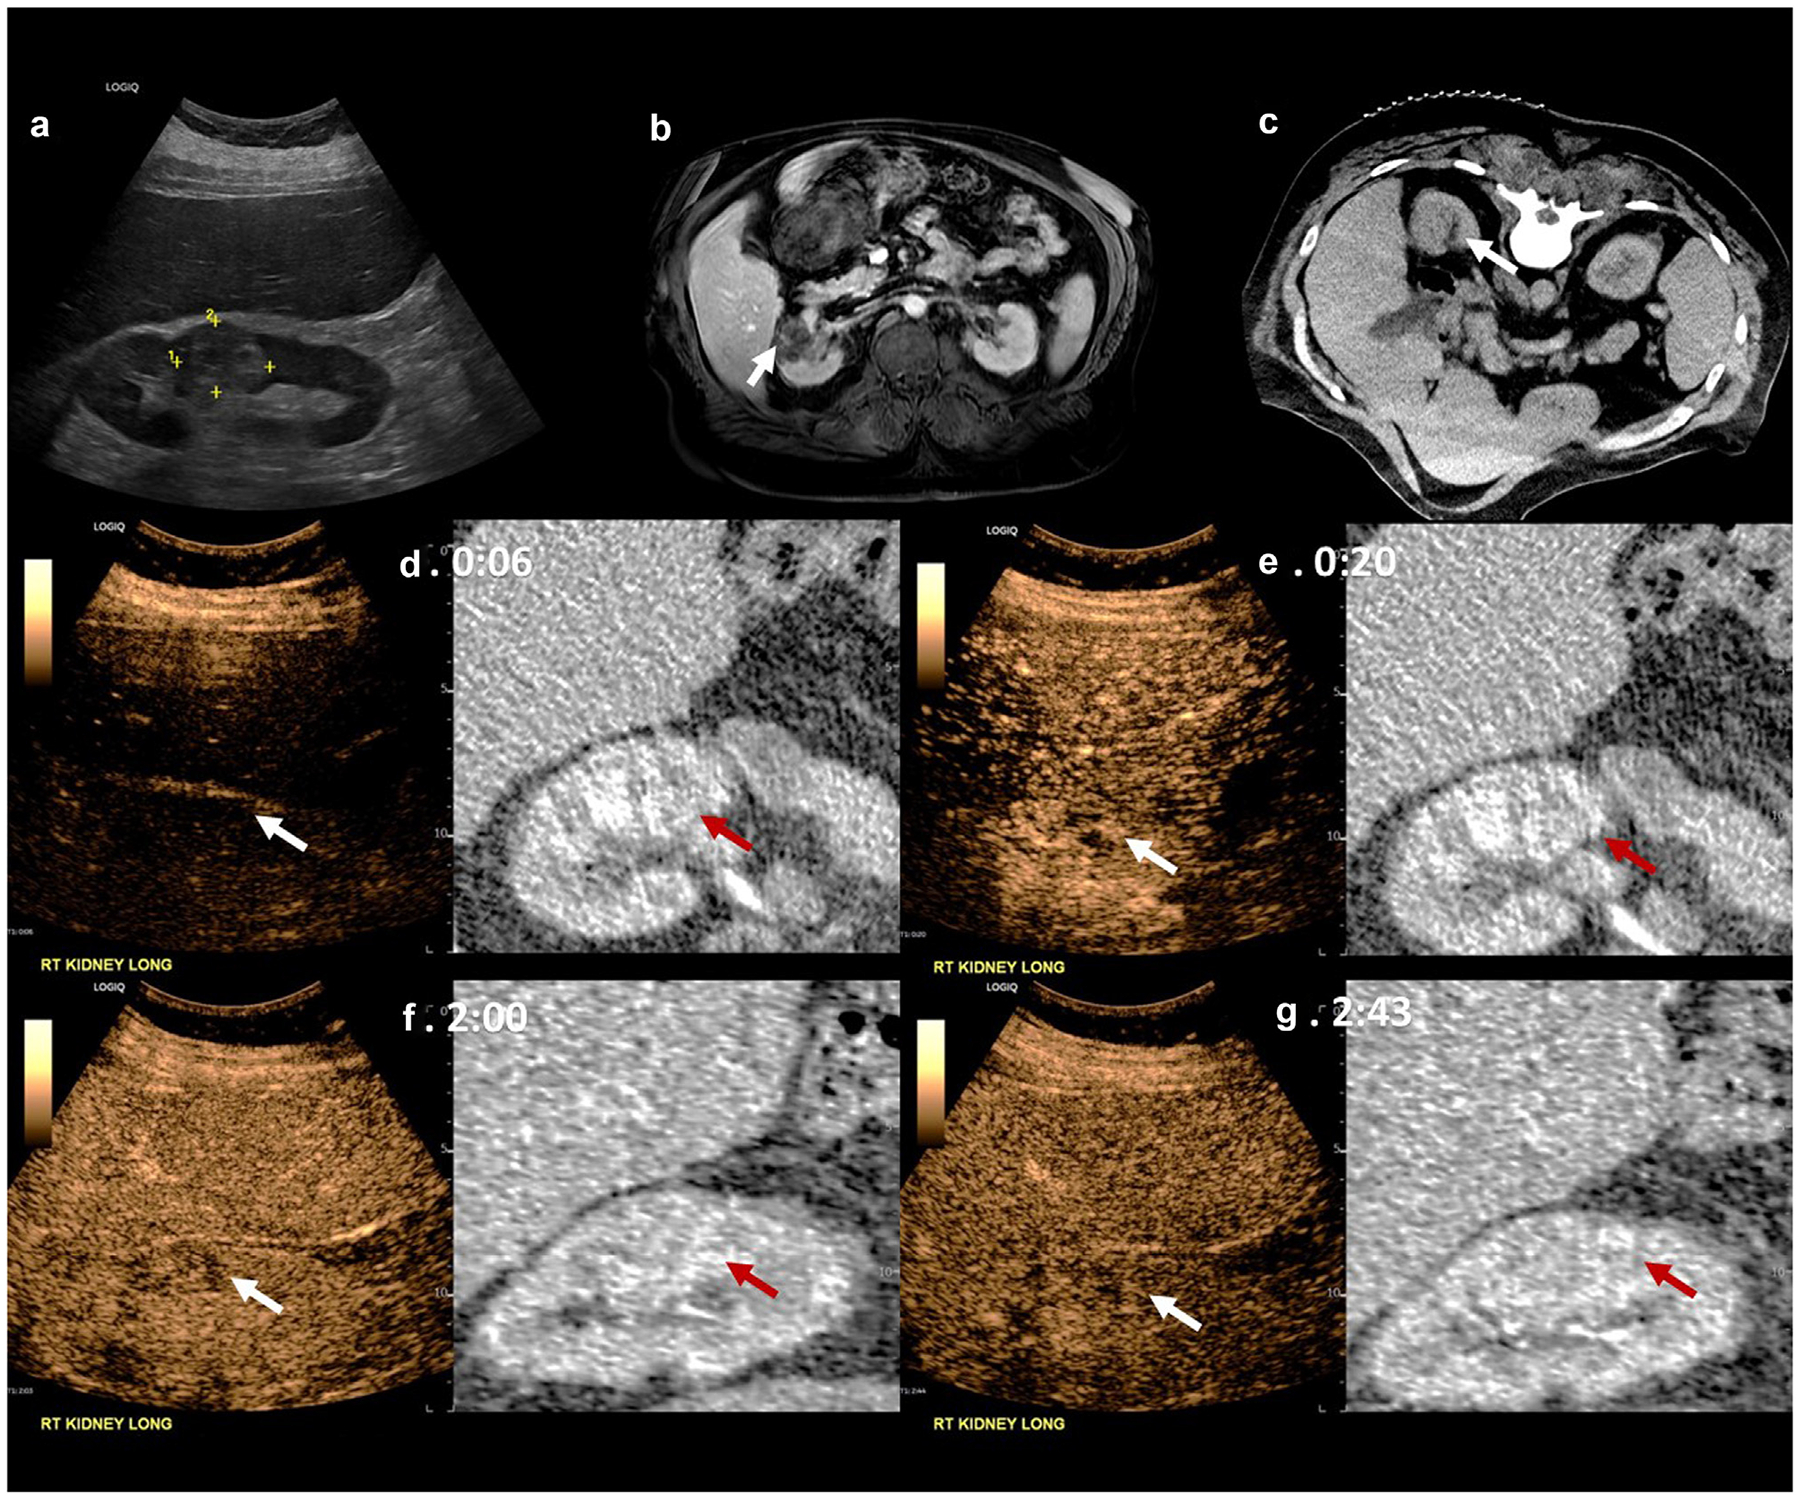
B-mode, MRI, CT and CEUS fusion imaging of a patient with disease recurrence. (a) Displays patient’s ultrasound B-mode image of the recurrent lesion in the previously ablated location with dimensions of 3.8 cm × 2.9 cm, (b) Patient’s most recent CE-MRI displaying the recurrent lesion (c) Displays patient’s preablation CT in, which was used for fusion imaging three years later Example of CEUS with fusion acquired (d) 6 seconds post-injection, (e) 20 seconds post-injection showing the arterial phase iso-enhancement, (f) 2 minutes post-injection at the start of wash out, and (g) 2 minutes and 43 seconds post-injection showing early mild washout.
